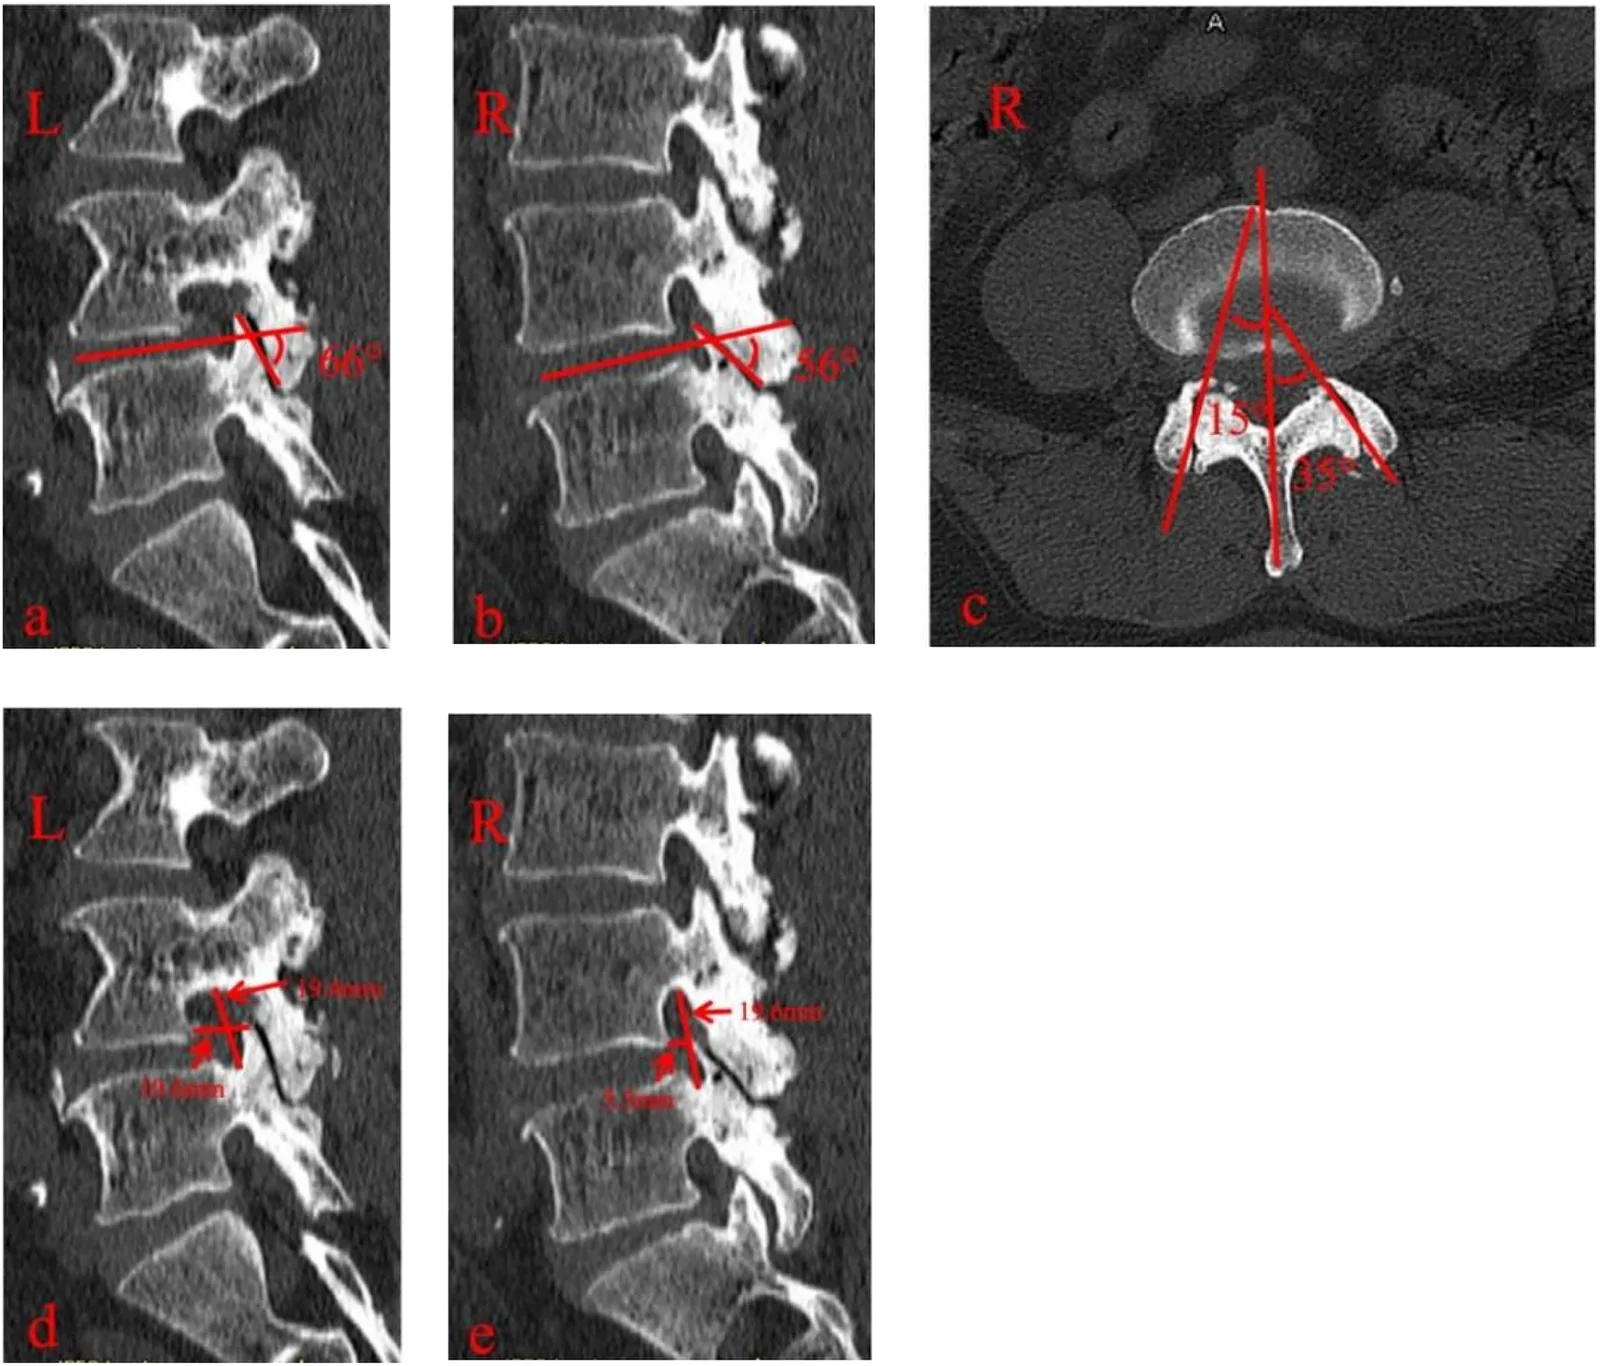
Preoperative CT scans demonstrated L4/5 facet joint degeneration. The difference of the bilateral facet joint angles was 10° in the sagittal plane (a,b) and 20° in the axial plane (c) The width of the right neuroforamen was smaller than that of the left, while the heights were nearly equivalent (d,e).
